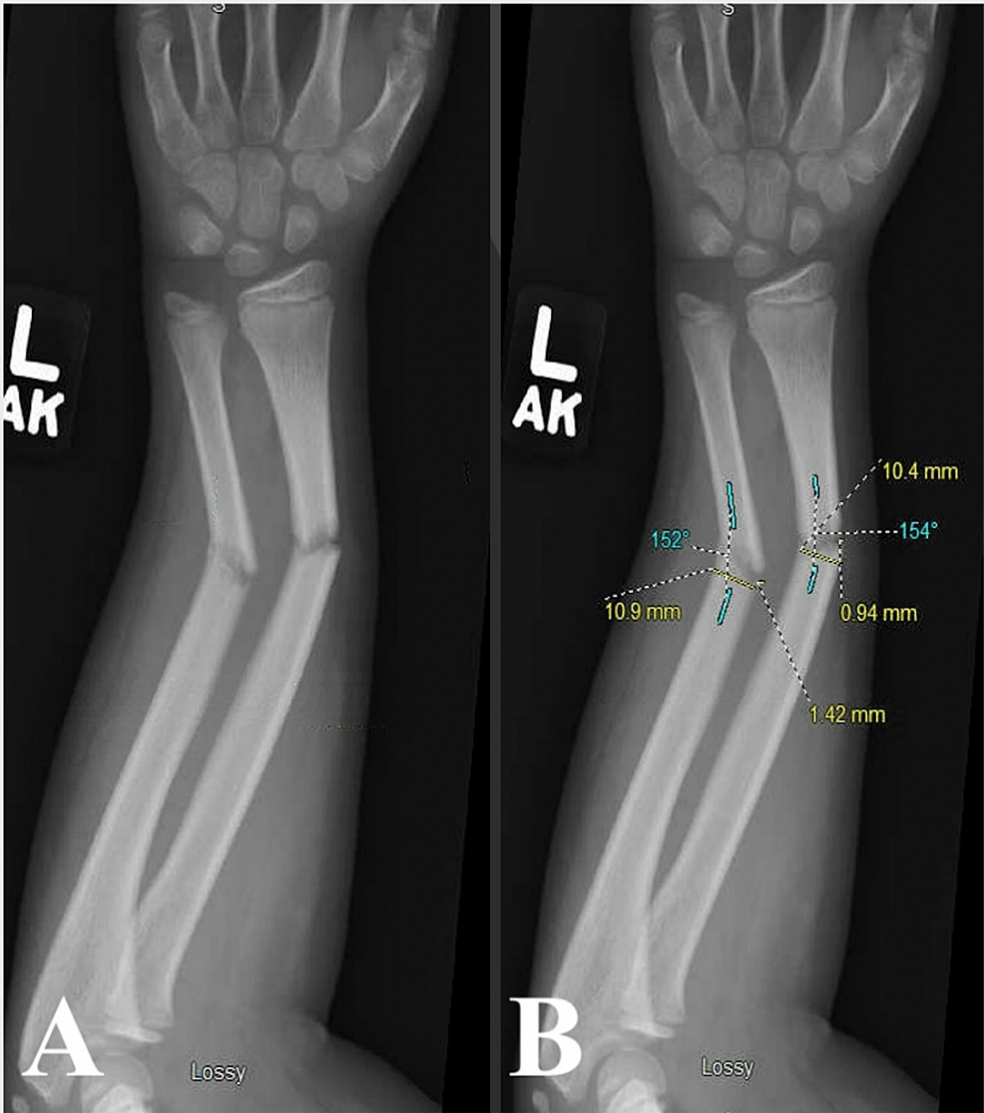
Pre-reduction radiograph in anteroposterior view with measurements unlabeled (A) and labeled (B). Yellow markings measure shaft radius and displacement, and blue markings measure the angle of deformity.
